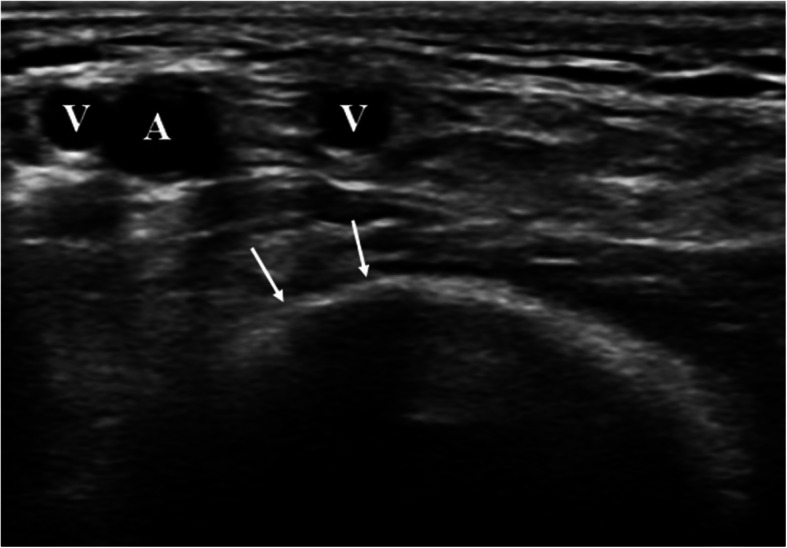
Transversal ultrasound picture of the A. brachialis (A) in the cubital fossa. The distal humerus (white arrows) forms an abutment, which helps for manual compressions. Two brachial veins (V) often accompany the distal A. brachialis
